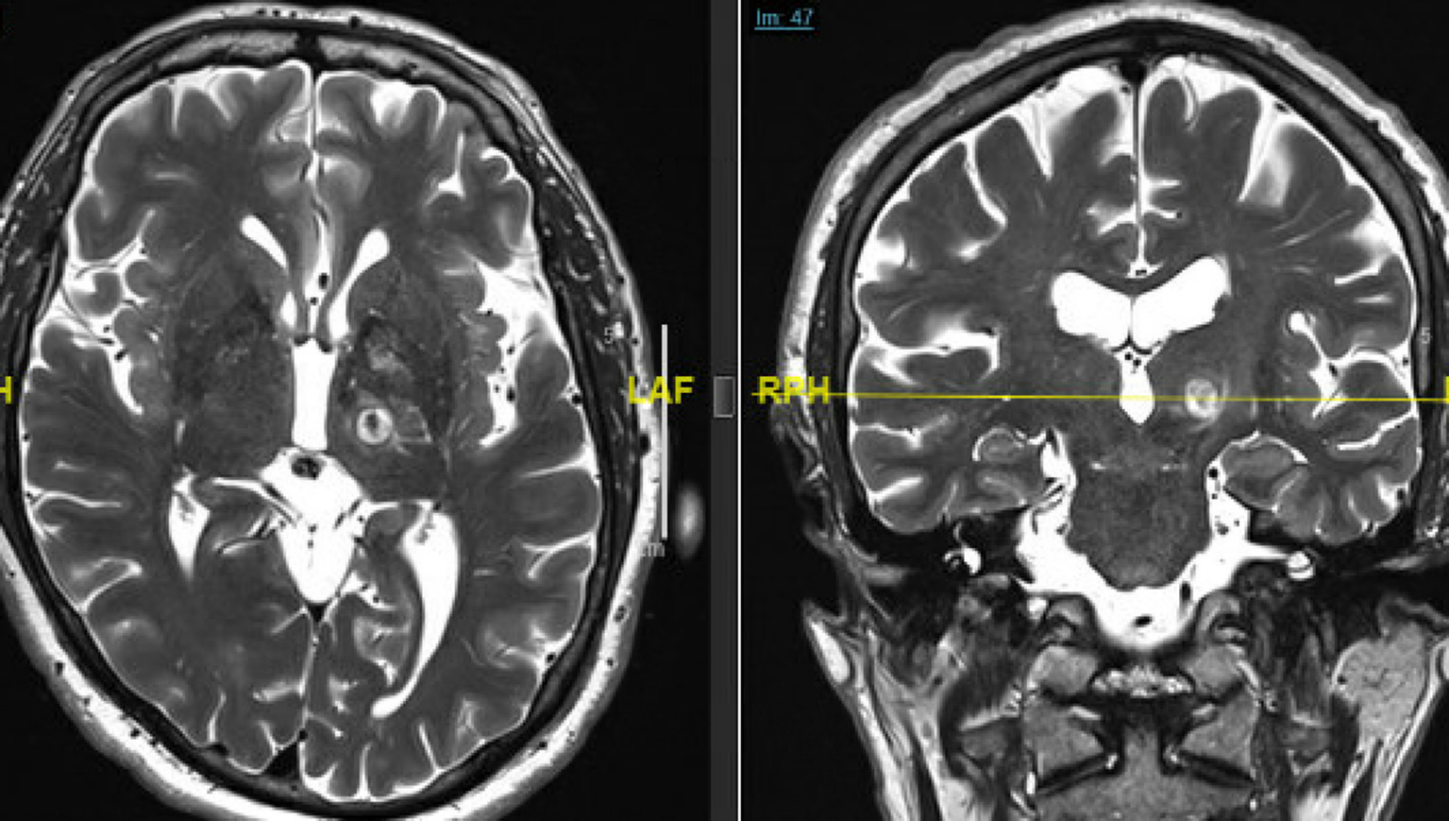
Immediate (24 h post-op) T2-weighted axial (left) and coronal (right) MRI scans demonstrating the size, shape, and location of a typical left Vim FUS thalamotomy. Note the edema extending around the lesion into the surrounding thalamus and the internal capsule.
